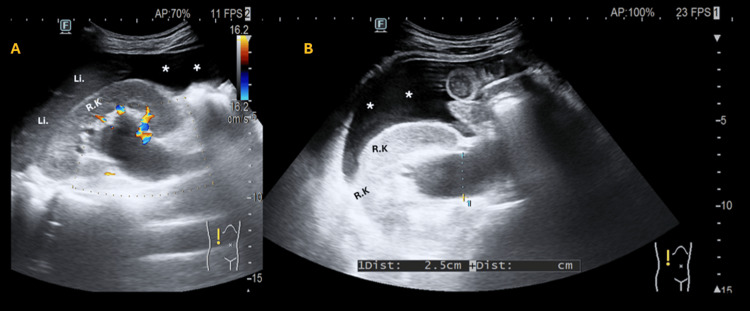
Abdominopelvic ultrasound on the right kidney (hydronephrosis): panels A and B. In panel A, absent Doppler flow confirms true pelvicalyceal dilatation. In panel B, the anteroposterior ureteropelvic diameter measures approximately 2.5 cm, a standard metric used for grading hydronephrosis.
